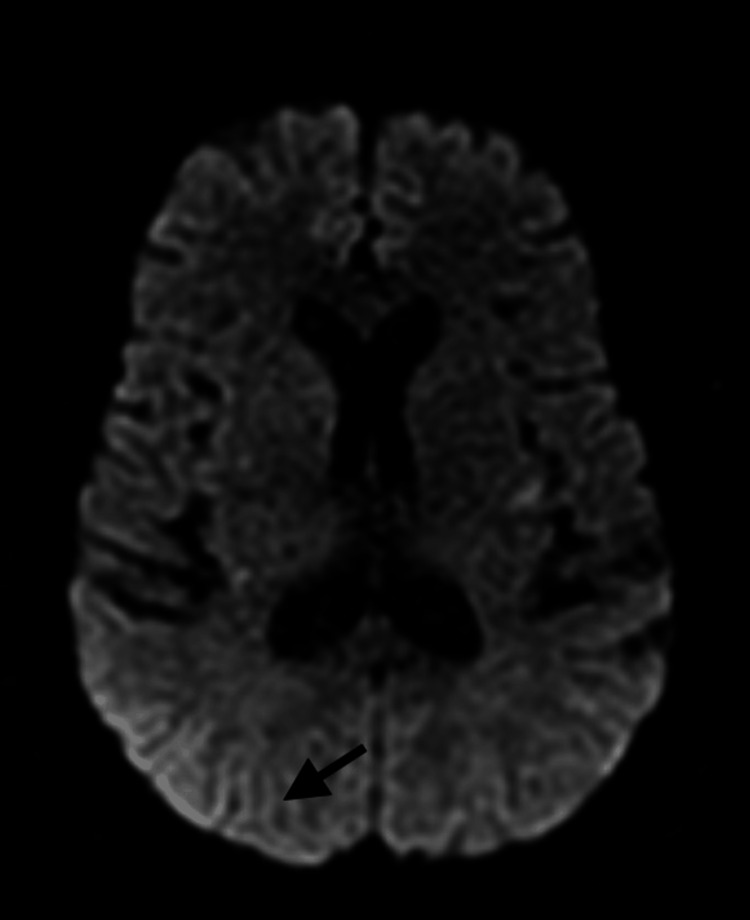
MRI brain showing multiple bilateral acute cerebral infarcts and a 0.9 cm acute intra-axial hemorrhage in the right parieto-occipital region (arrow)
